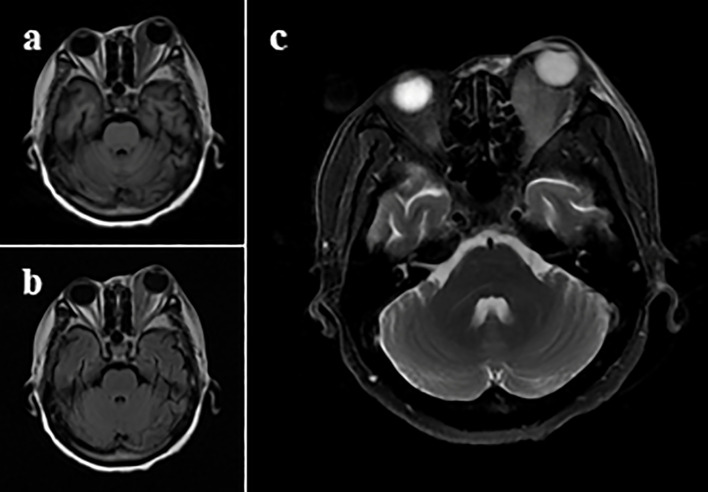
MRI of the head. T1 FLAIR (a), T2 FLAIR (b), and T2 FS FRFSE (c). Images showed abnormal hyperintensity and enlargement of the left extraocular muscles (medial, superior, and inferior rectus), with associated mild proptosis, suggestive of an inflammatory pseudotumor.
